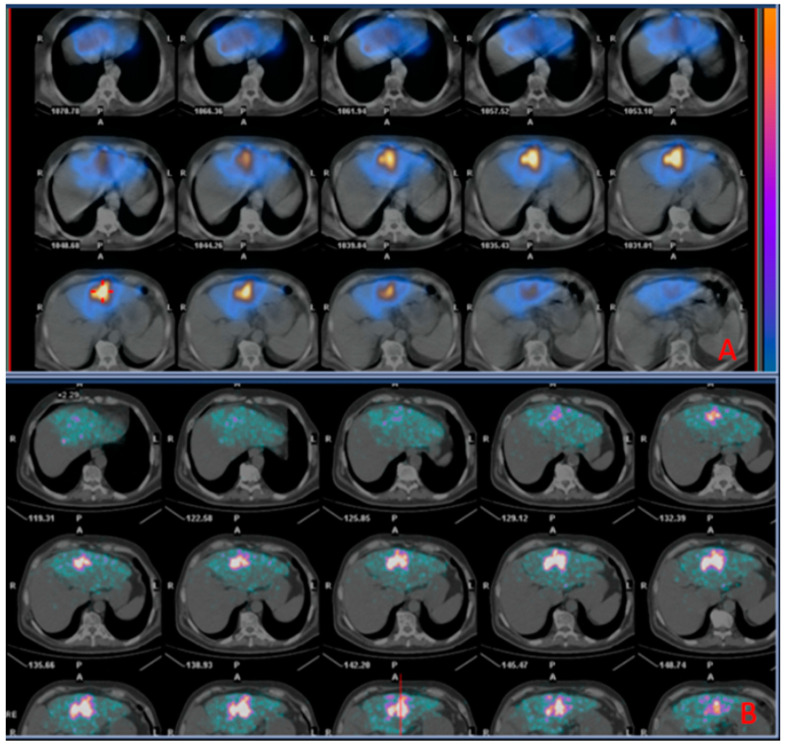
Images of a patient undergoing radioembolization of a hepatic metastasis from colorectal cancer. (A) (Upper box): SPECT/CT images (diagnostic phase) 2 h acquired after administration of ^99m^Tc-MAA; (B) (Inferior box): PET/CT images (therapeutic phase) acquired 2 h after the administration of ^90^Y TheraSpheres, showing successful distribution of ^90^Y TheraSpheres matching that of ^99m^Tc-MAA in the diagnostic phase.
